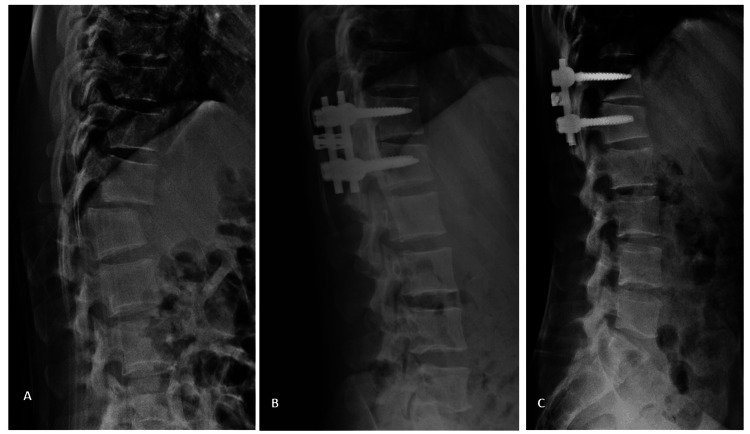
A 32-year-old male with AO type C D12–L1 injury. (A) Preoperative radiograph showing the dislocation. (B) Postoperative radiograph following reduction and mono-segment fixation. (C) Postoperative radiograph at one year demonstrating intact fixation.
